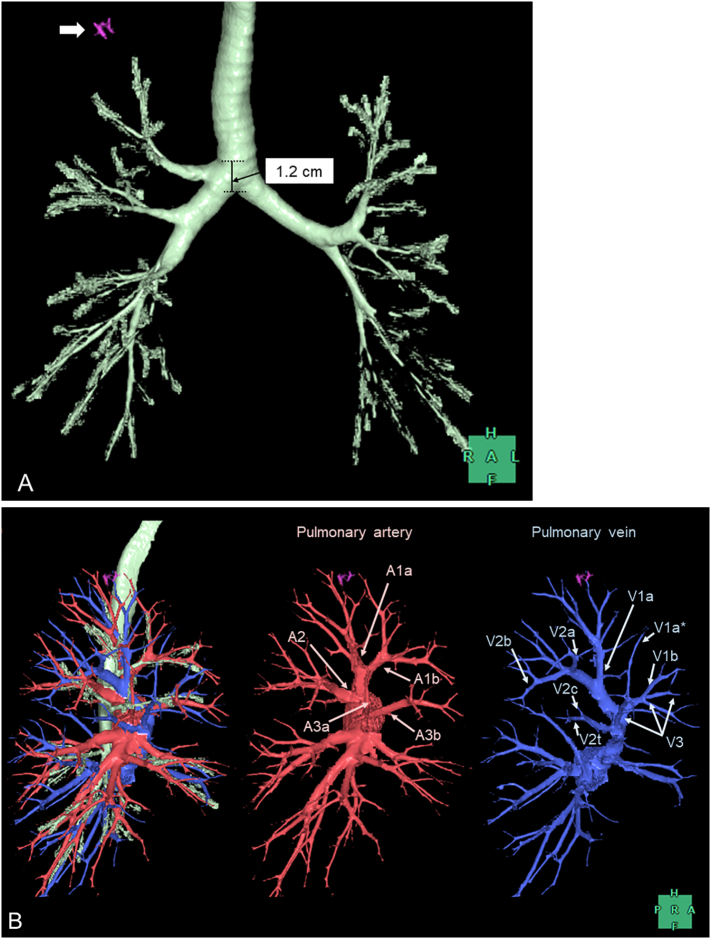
Three-dimensional computed tomography image. (A) The tracheal bronchus of the right apical segment branches from the trachea within 1.2 cm above the carina. The pink nodule (white arrow) is the tumor. (B) The pulmonary vein runs ventral to the pulmonary artery. The superior trunk (A1) independently branches from the main pulmonary artery. V1a and V2a + b share a common trunk that drains to the anterior vein, while the small branch of V1a (V1a*) drains to V1b.
